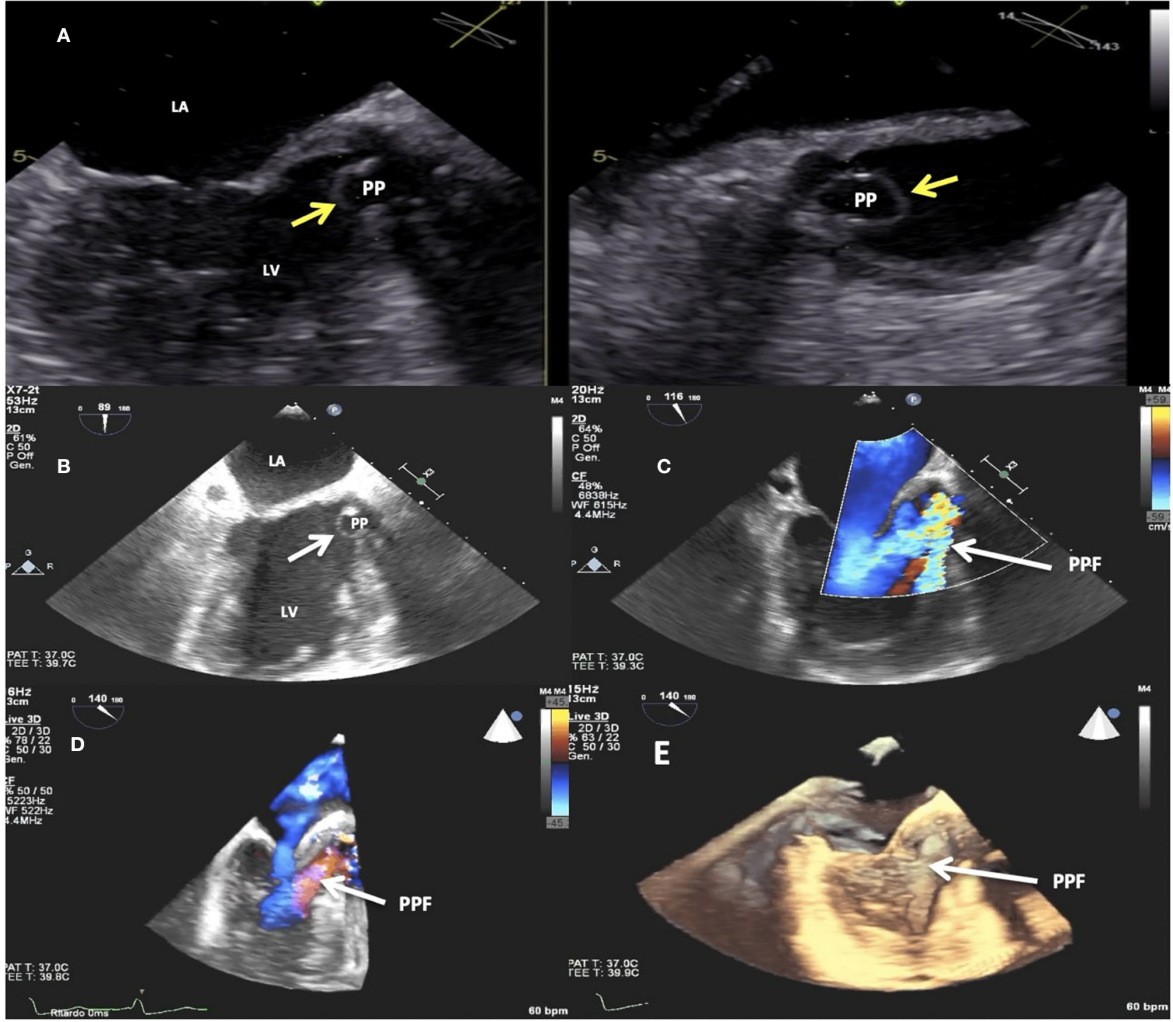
Transesophageal echocardiogram (TEE). 2D imaging showing the paravalvular pseudoaneurysm (yellow arrows) protruding in diastole into the left ventricle outflow tract (A,B). 2D (C) and 3D (D,E) TEE color Doppler showing the paravalvular pseudoaneurysm (white arrows) protruding in diastole into the left ventricle outflow tract and the paravalvular pseudoaneurysm fistula causing severe regurgitation. LV, left ventricle; LA, left atrium; PP, paravalvular pseudoaneurysm; PPF, paravalvular pseudoaneurysm fistula.
